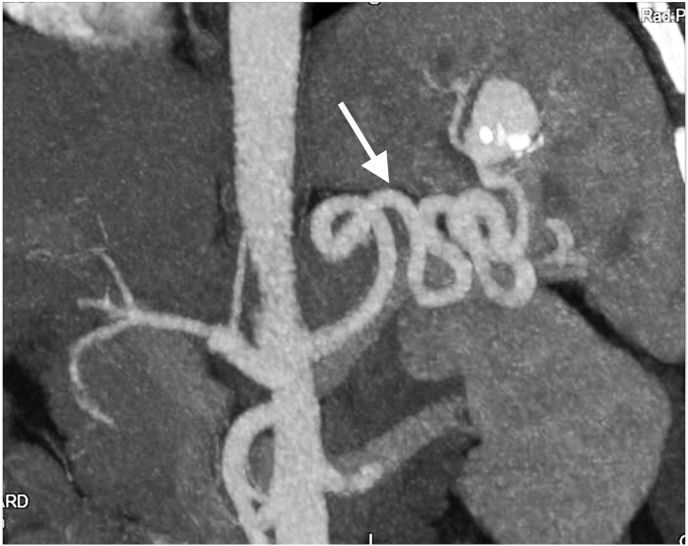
Coronal CT. White arrow showing splenic arterial aneurysm.
